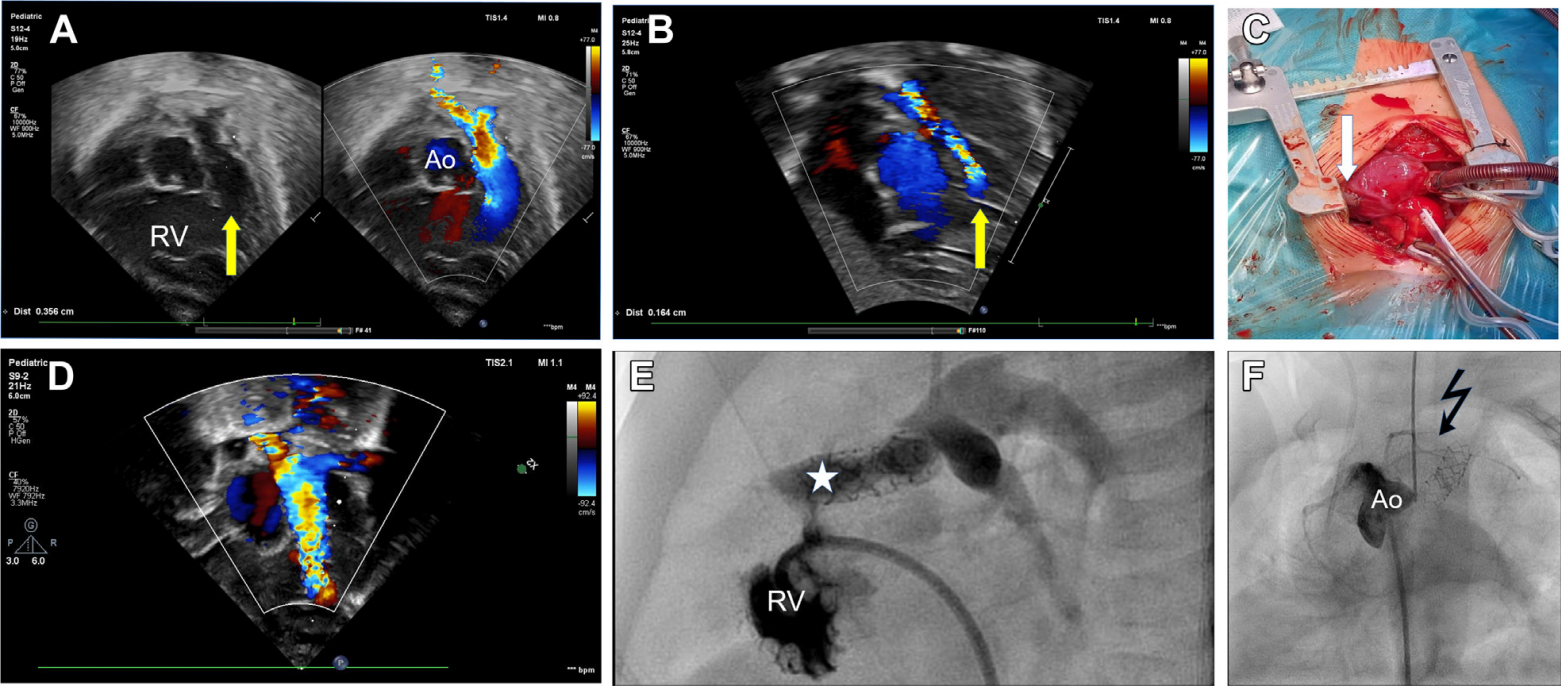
Progressive Right Outflow Tract Obstruction Despite Stent Implantation (A) Transthoracic echocardiogram (TTE). Subcostal view with color Doppler flow showing mildly stenotic right ventricular outflow tract (yellow arrow) on the first day of life with effective pulmonary flow. (B) TTE. Subcostal view with color Doppler flow showing severely stenotic right ventricular outflow tract (yellow arrow) impairing pulmonary flow. (C) Intraoperative view of coronary arteries with wide additional left anterior descending artery crossing outflow tract and a right ventriculotomy patch (white arrow) for hybrid procedure. (D) TTE. Subcostal view with color Doppler flow showing effective RV outflow tract flow through the implanted stent. (E) Right ventriculography in lateral view showing severe intraventricular stenosis by hypertrophied muscular bands obstructing the flow through the implanted stent (white star). (F) Aortography (CAU 40, CRA 30) showing right coronary artery, additional left anterior descending artery, and conal branch crossing outflow tract (dark jagged arrow). Ao = aorta; CAU = caudal; CRA = cranial; RV = right ventricle.
